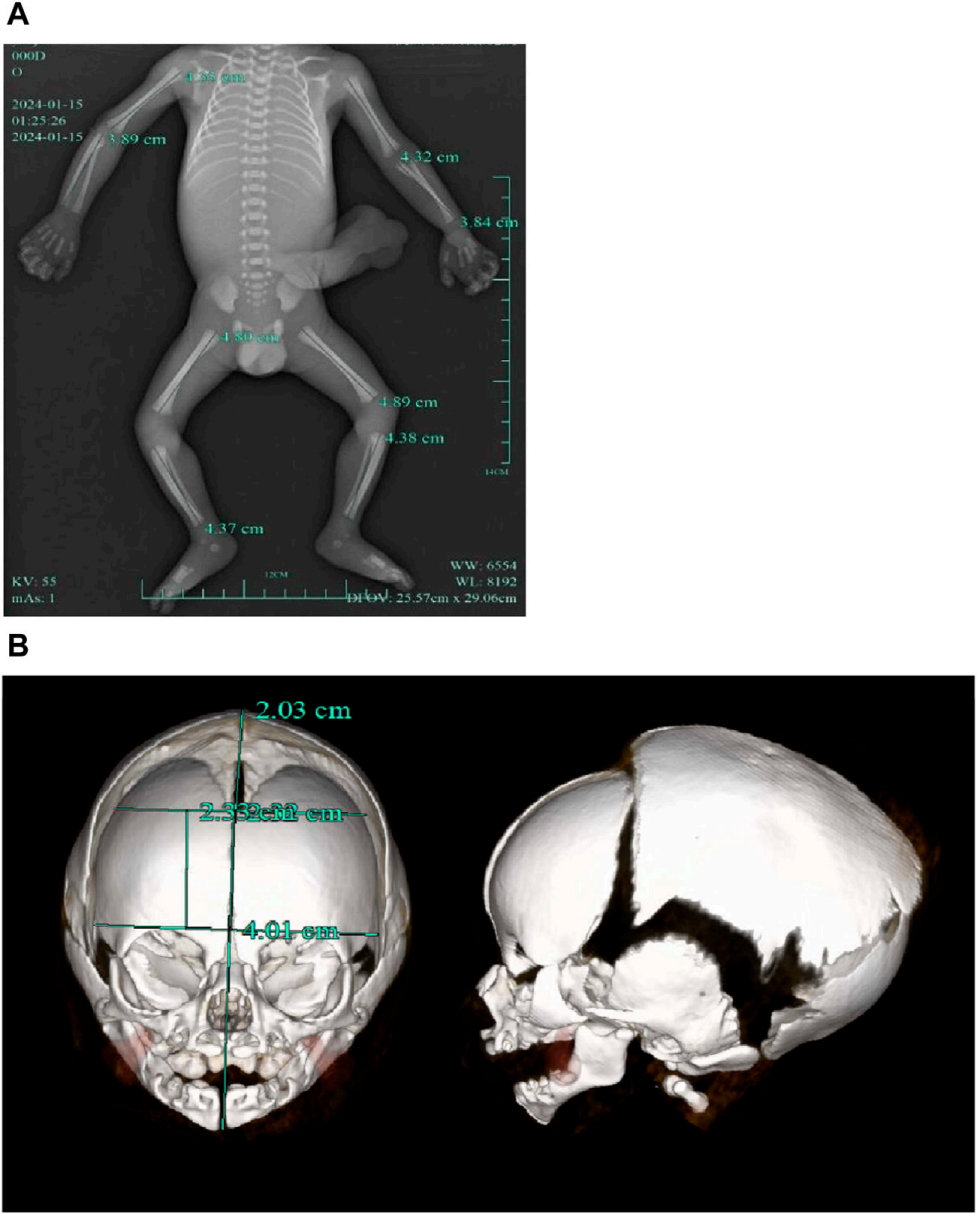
(A) CT scan of trunk and extremities did not show body asymmetry. (B) The head CT scan showed relative macrocephaly and micrognathia.
